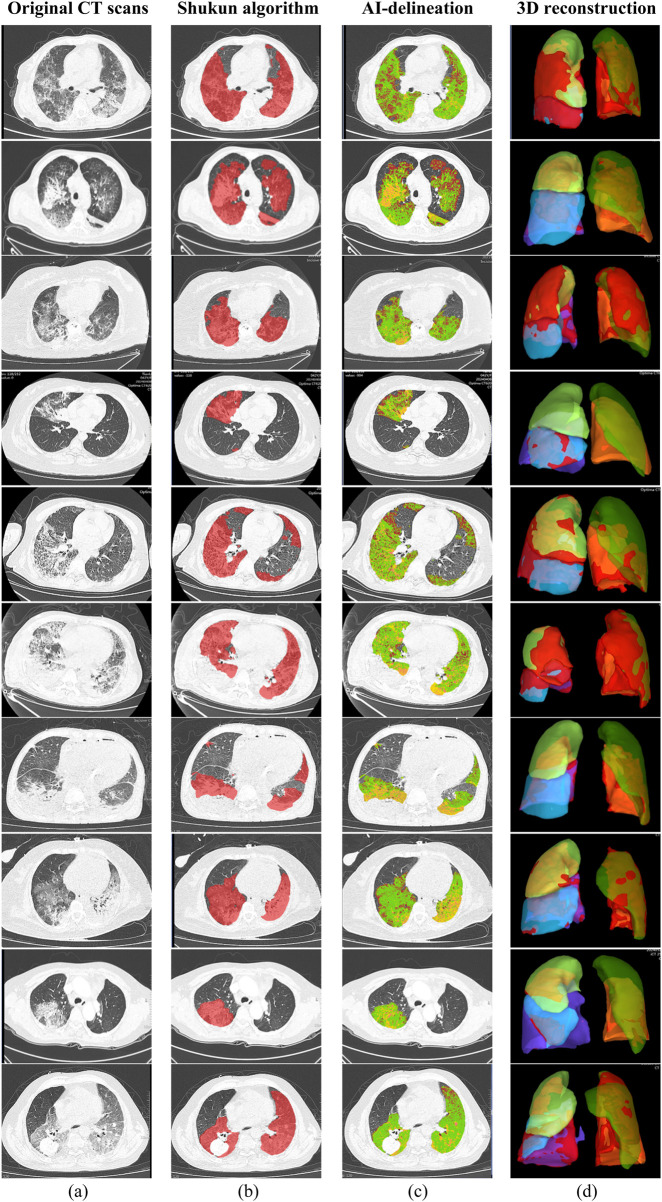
Chest CT images of 10 sample patients with pneumonia; (a) Original CT scans; (b) Pneumonia lesions delineated using the Shukun AI algorithm; (c) AI-delineated lesions with green indicating ground-glass opacities and yellow indicating consolidation; (d) 3D reconstruction of the lungs, with red indicating pneumonia lesions.
